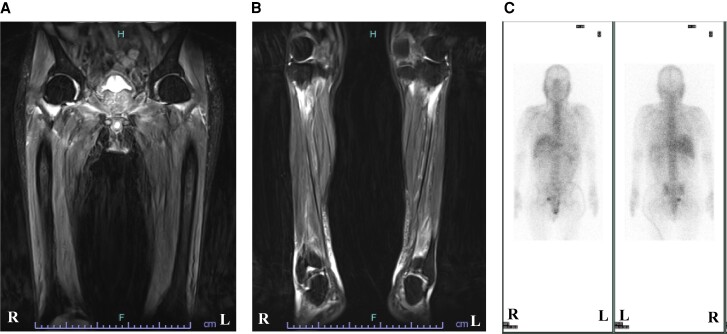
Follow-up T_2_-weighted magnetic resonance imaging of the lower limbs and gallium scintigraphy. The follow-up magnetic resonance imaging on Day 38 revealed the absence of high signal intensity lesions in both the proximal (*A*) and distal muscles (*B*). Gallium scintigraphy on Day 45 did not show any visible accumulation of gallium (*C*).
